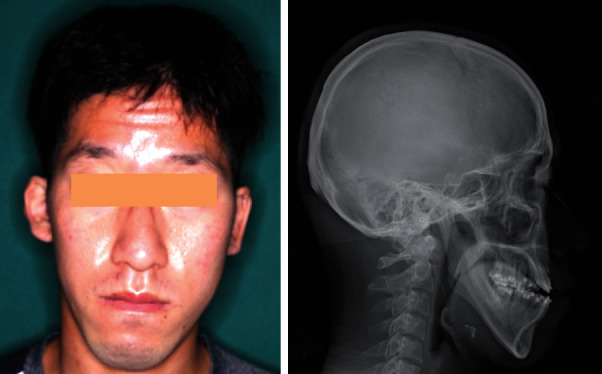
Preoperative facial photograph and lateral cephalometric radiograph. The mandible is deviated to the right, with anterior crossbite.
